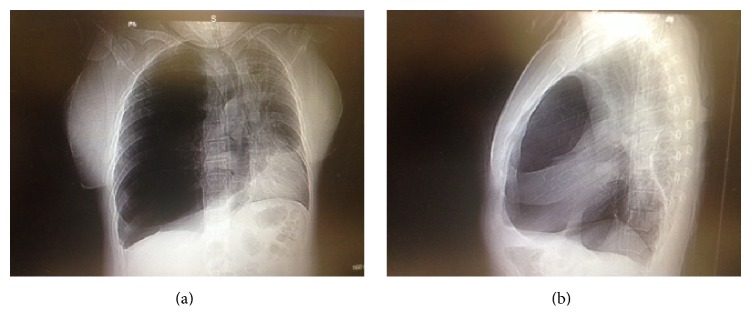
Posteroanterior (PA) chest radiograph depicts an increased translucency of the whole right hemithorax and also severe lateral displacement of the mediastinal structures (a). In addition, the lateral view shows the hyperinflated lobe displacing the oblique fissure and also displacing the mediastinum (b).
